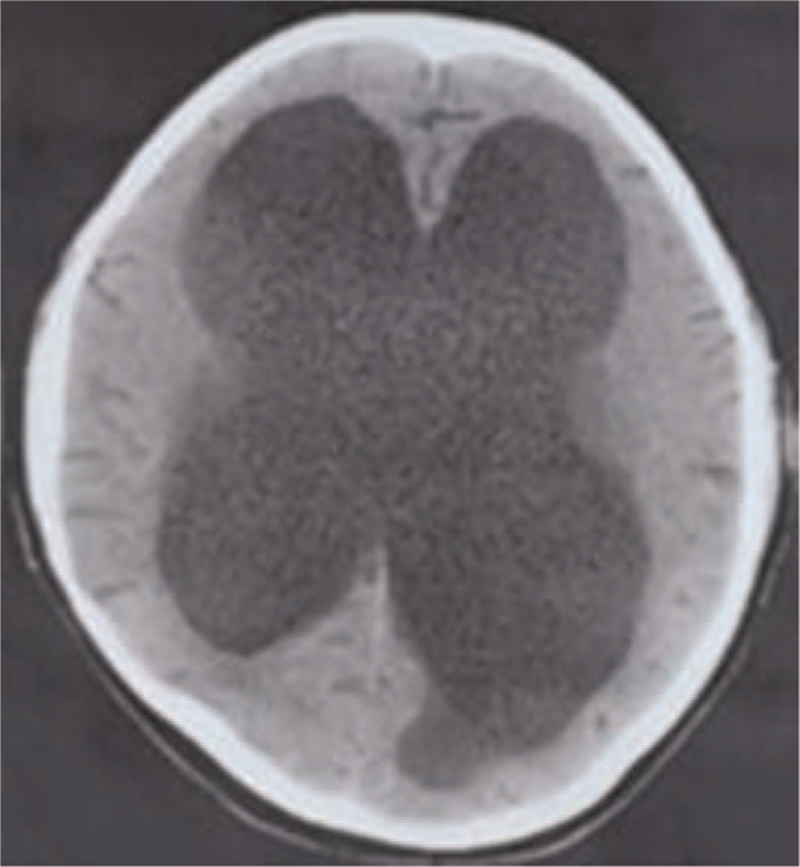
CT of the local hospital demonstrated that evident increase in ventricular size and reduction of sulci at parenchymal level.
